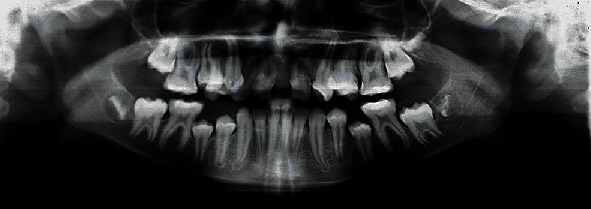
Pretreatment pantomogram X-ray.
